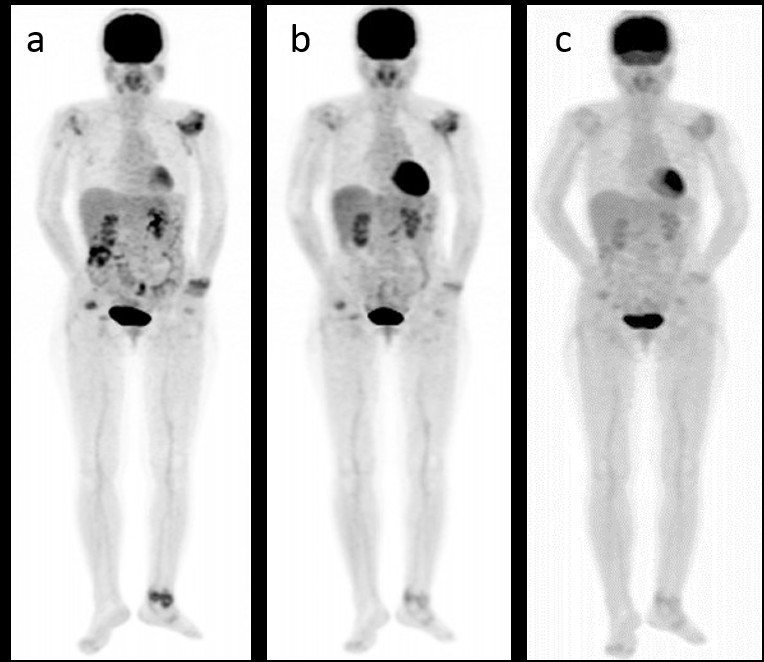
Images of FDG-PET obtained under TCZ therapy. (a) Whole-body ^18^F-FDG-PET/CT was performed before (a) at three (b) and six months (c) after the initiation of TCZ therapy. The FDG uptake in the affected joints had decreased. TCZ: tocilizumab. The contrasts of the PET images were adjusted on the basis of the liver density.
